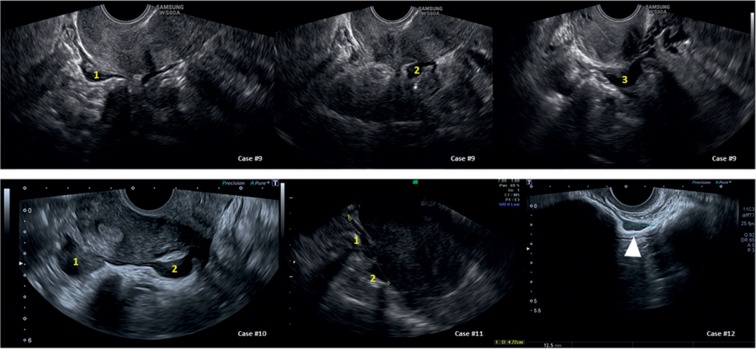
Differential diagnosis for bowel endometriosis (sonographic details of each case are reported in Table 2).
